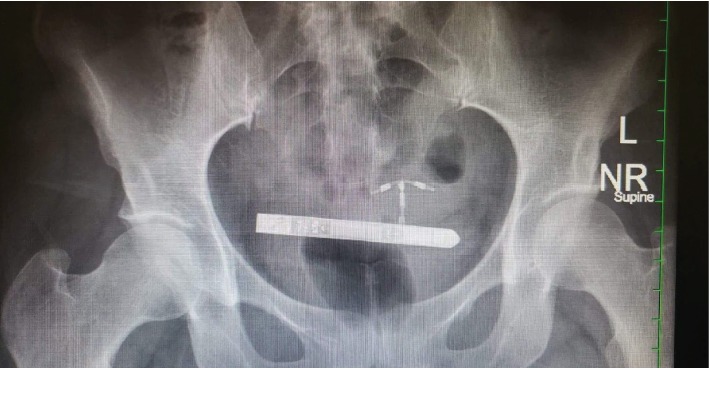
Abdominal and pelvic X-ray showing the device within the pelvis.
